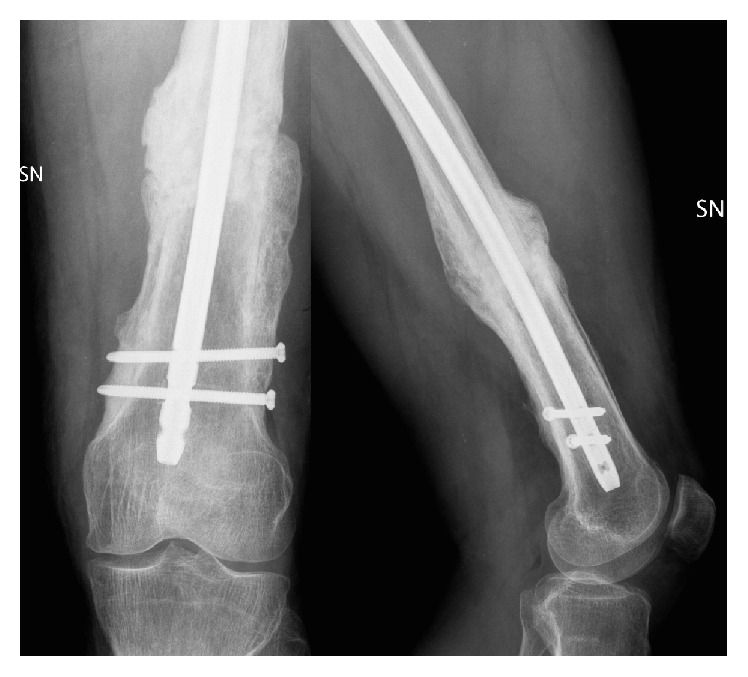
At 20 months of follow-up, the radiographic examination showed the complete healing of the femoral shaft nonunion.
